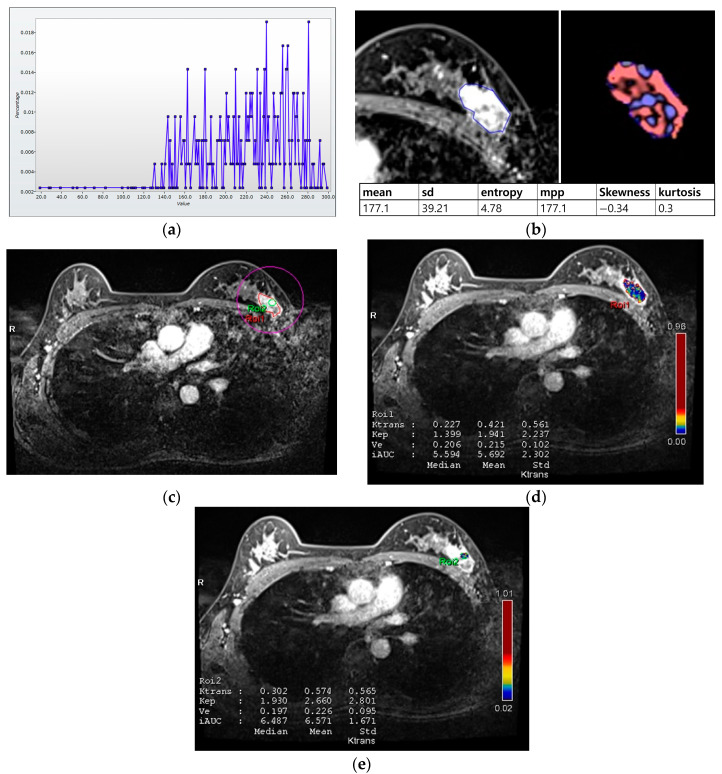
Histogram and perfusion analyses conducted on breast MRI in a 42-year-old woman with a 30 mm triple-negative invasive ductal carcinoma of the left breast (a,b). Histogram analysis on MRI: Axial contrast-enhanced T1-weighted MRI image shows an oval shaped, irregular marginated, and heterogeneous enhancing mass in the left breast. The region of interest (ROI) was drawn manually for the entire tumor, and a histogram was obtained (a). From the histogram, six statistically based metrics were extracted: mean, standard deviation (SD), mean of positive pixels (MPP), entropy, skewness, and kurtosis (b). (c–e) Perfusion analysis on MRI: Two ROIs (ROI1: entire tumor and ROI2: hot spot) were manually delineated (c), and eight perfusion parameters from each ROI were obtained: median and mean values of Ktrans, kep, Ve, and iAUC (d,e).
